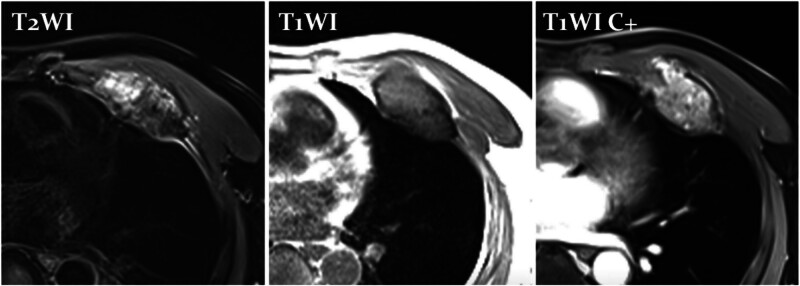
Magnetic resonance imaging showed conventional chondrosarcoma on MRI showed medium-low signal on T1WI and uneven high signal on T2WI. MRI = magnetic resonance imaging.
